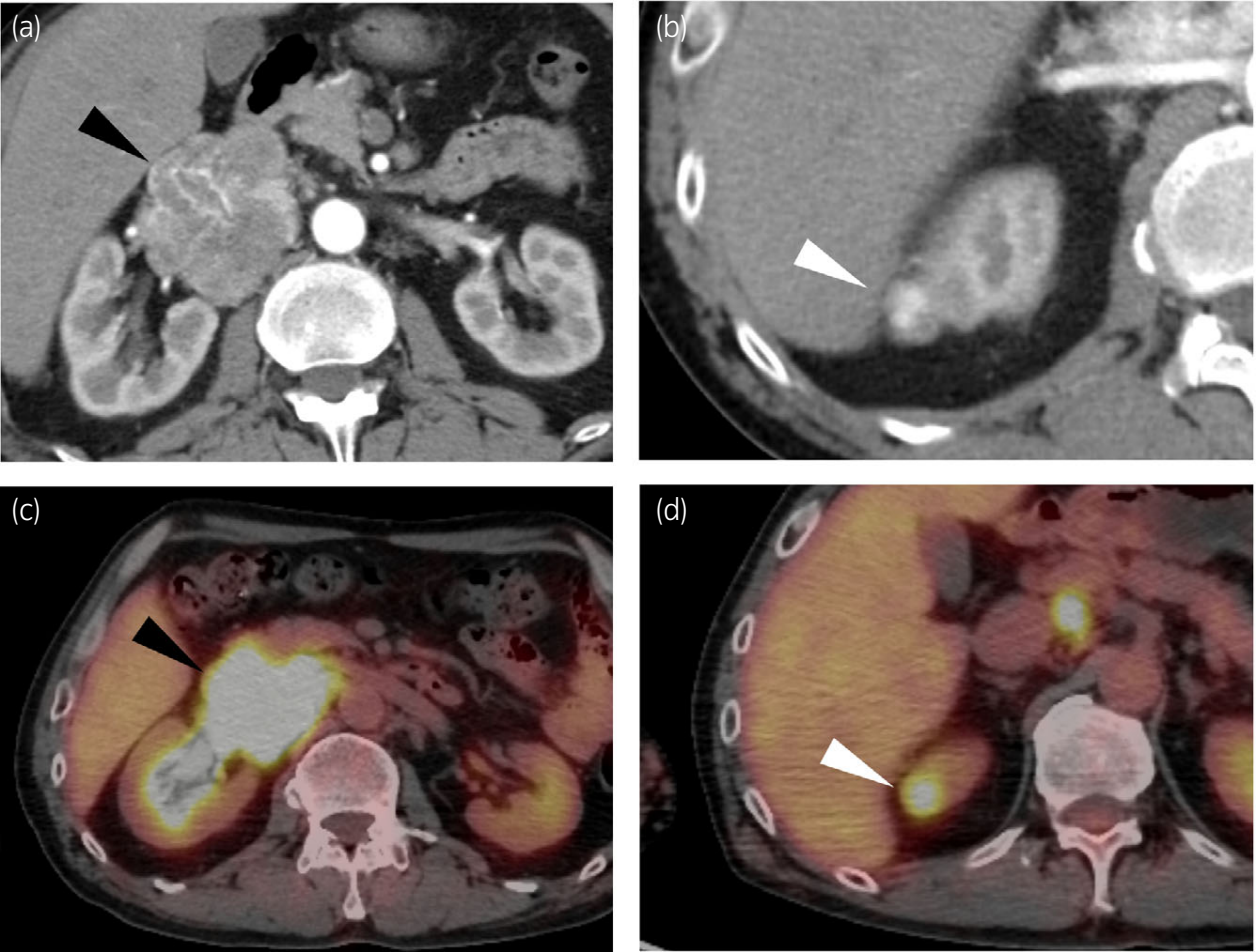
Radiological findings. Contrast‐enhanced CT images of (a) retroperitoneal paraganglioma (black arrowhead) and (b) right renal tumor (white arrowhead); ^18^F‐FDG‐PET images of (c) retroperitoneal paraganglioma (black arrowhead) and (d) right renal tumor (white arrowhead).
